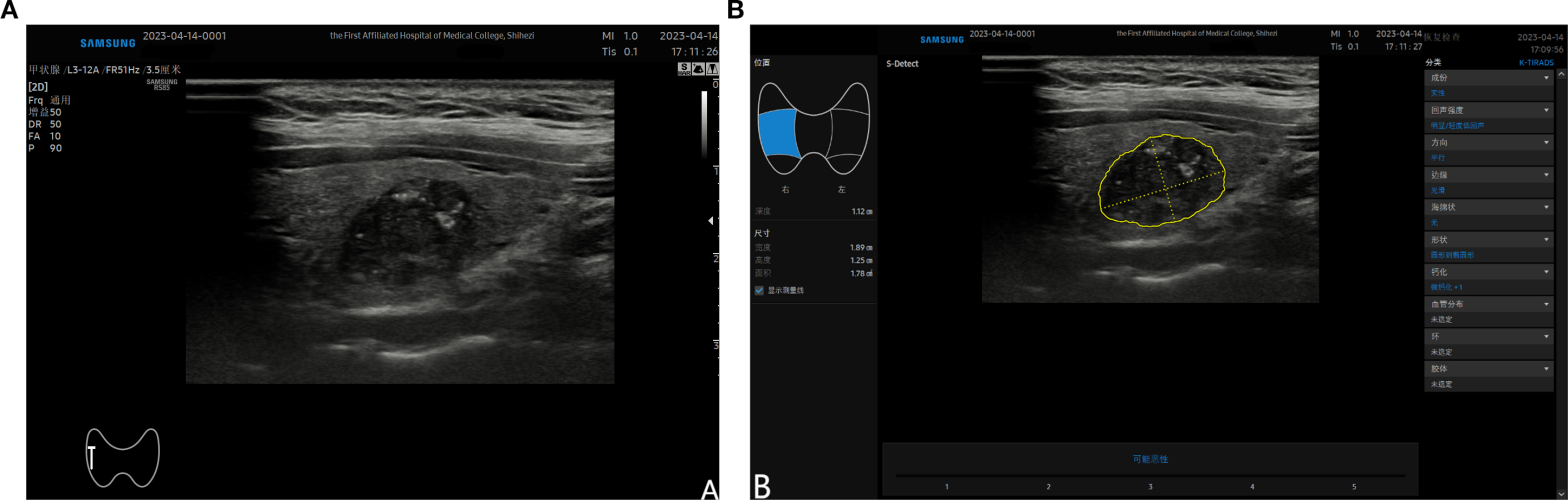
(A) shows a malignant nodule located in the mid-section of the right lobe of the thyroid detected by ultrasound; (B) demonstrates the automatically outlined nodule area after entering the S-Detect mode and selecting the “auto tracing” option. The nodule characteristics are displayed on the right side of the screen, and the analysis results are shown at the bottom.
